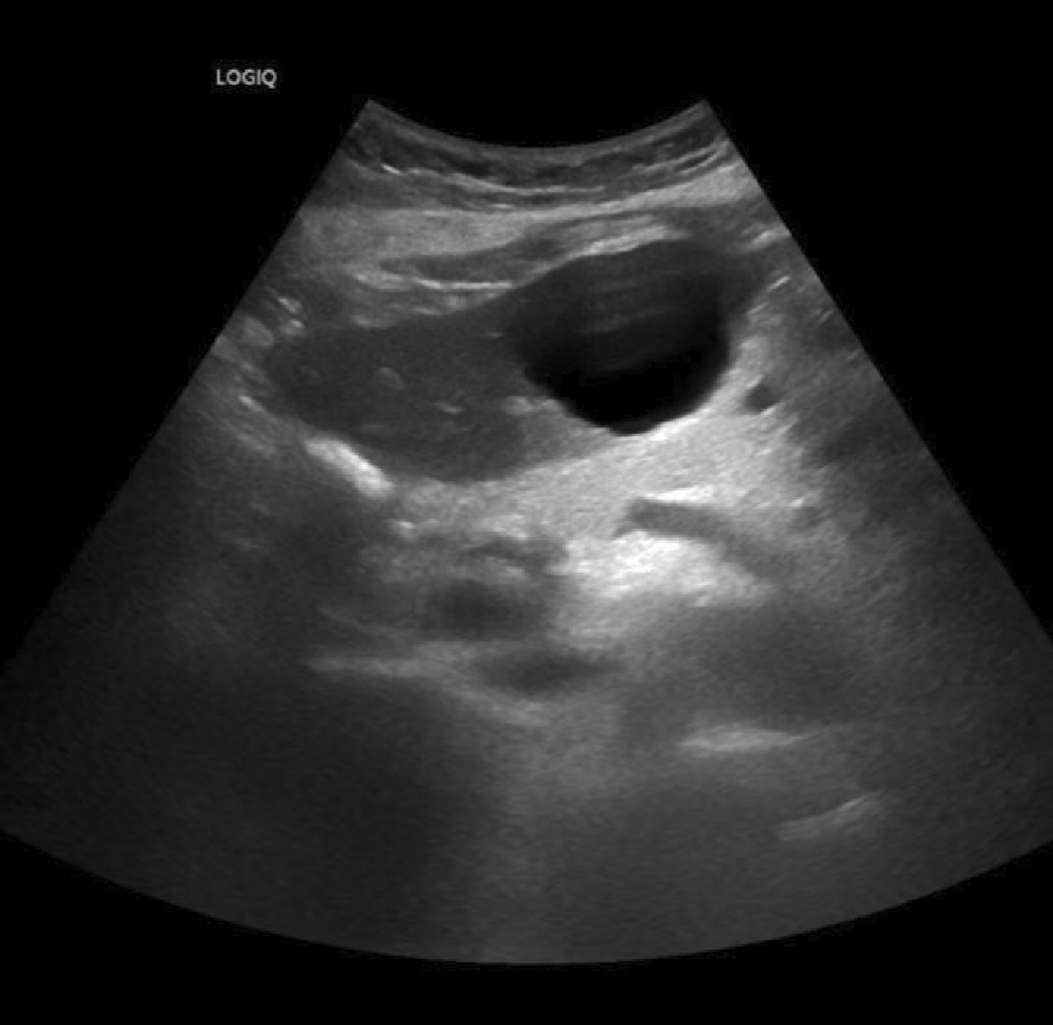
Ultrasound image showing a hypoechoic hepatic cyst (Ø 43 mm) in the left lobe.
